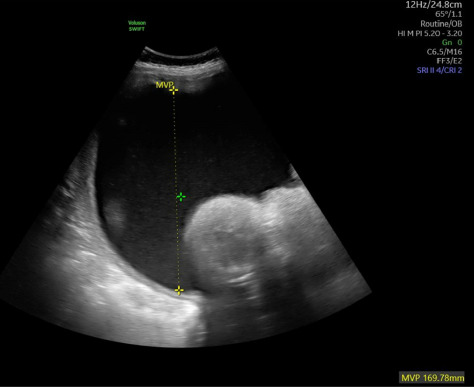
Ultrasound demonstrating severe polyhydramnios, with a maximum vertical pocket of 16.9 cm.
